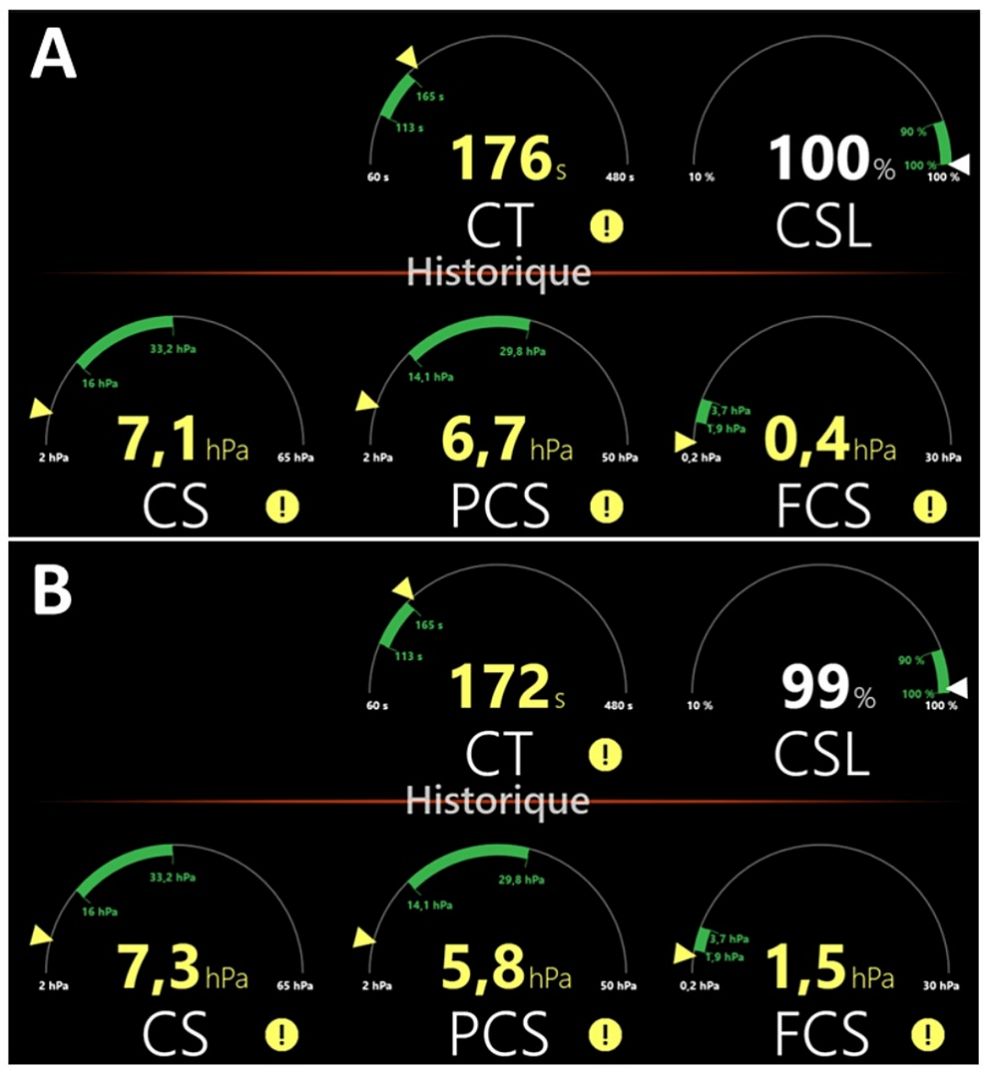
Viscoelastic hemostatic status of the patient evaluated using the Quantra QStat® system. Whole blood measurements were performed (A) two hours and (B) seven hours after placenta delivery. Parameters obtained with Quantra QStat® cartridge include clot time (CT), clot stiffness (CS), fibrinogen contribution to clot stiffness (FCS), platelet contribution to clot stiffness (PCS), and clot stability to lysis (CSL). Normal reference ranges are indicated by the green areas.
